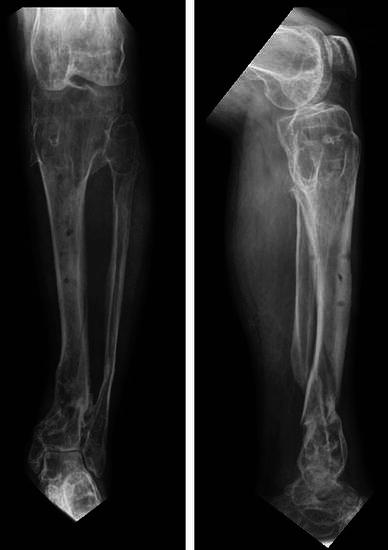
Radiographic position following solid union
